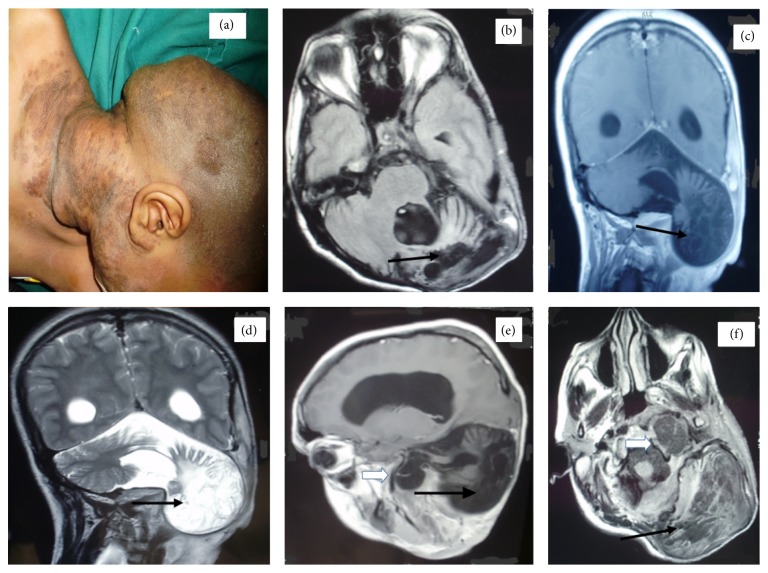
Clinical photo of the plexiform neurofibroma and cafe au lait spots (a). Axial T1 (b, f), coronal T1 (c), coronal T2-weighted (d), and sagittal T1-weighted (e) MR images showing occipital meningoencephalocele (black arrow) and parapharyngeal meningocele (hollow arrow).
